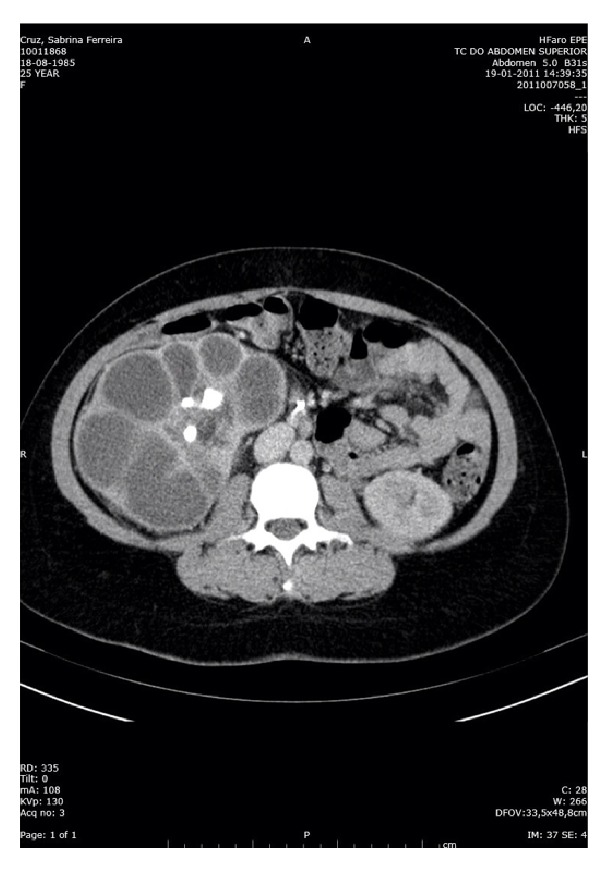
Abdominal pelvic CT: presence of a dilated collecting system with the presence of a coraliform calculus of 55 mm in the renal pelvis.
